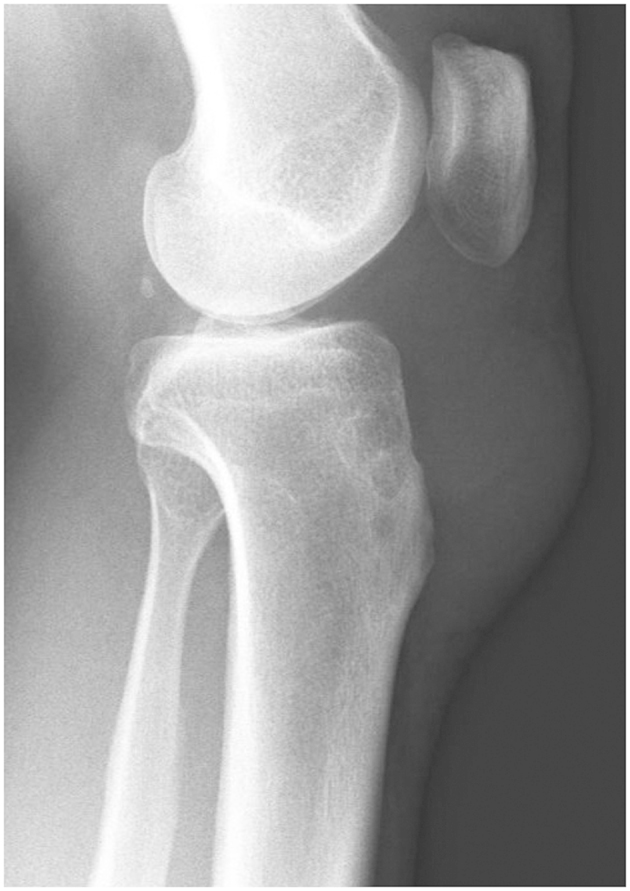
Plain lateral radiograph of the left knee demonstrating erosion of the tibia.
